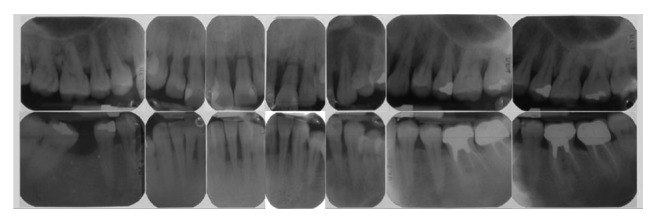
Intraoral periapical radiographs showing horizontal bone loss at all four maxillary incisors.
